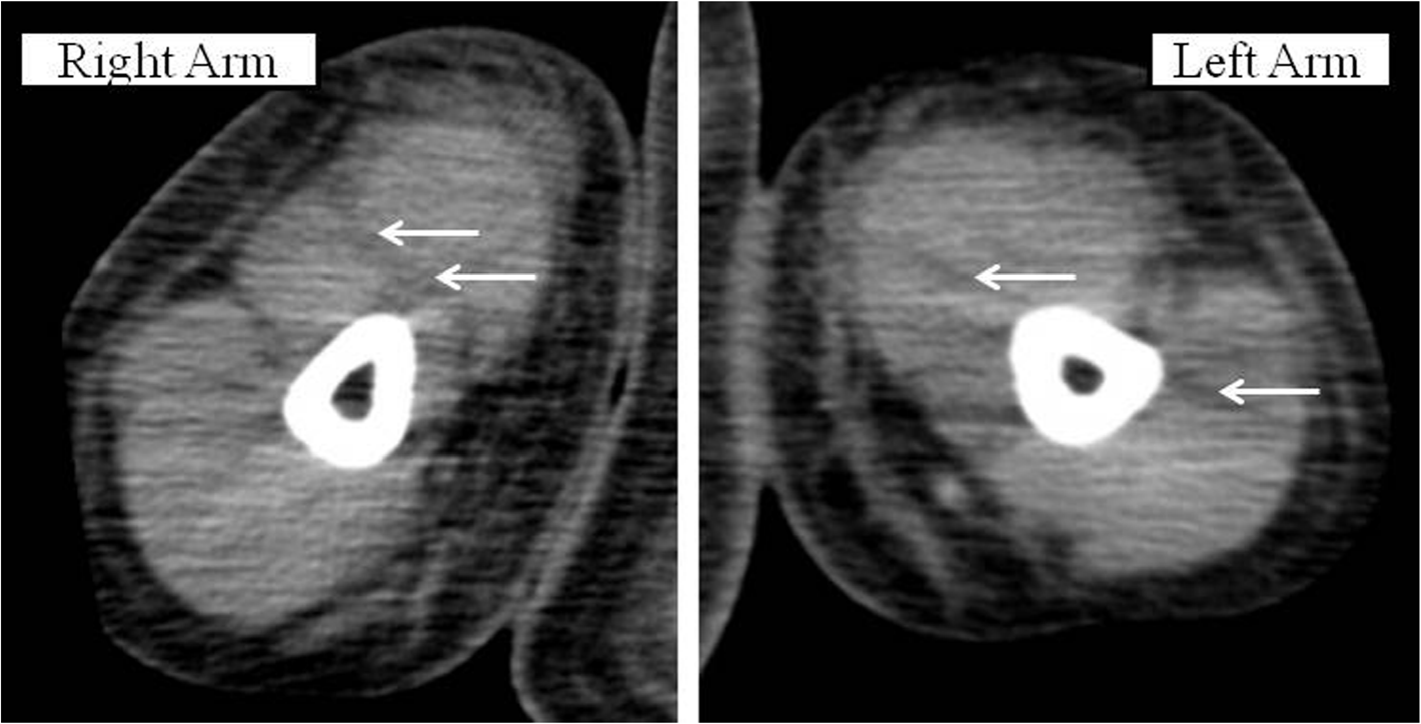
Computed tomography images of the forearms. Non-contrast CT images show edema between the muscles (white arrows)
